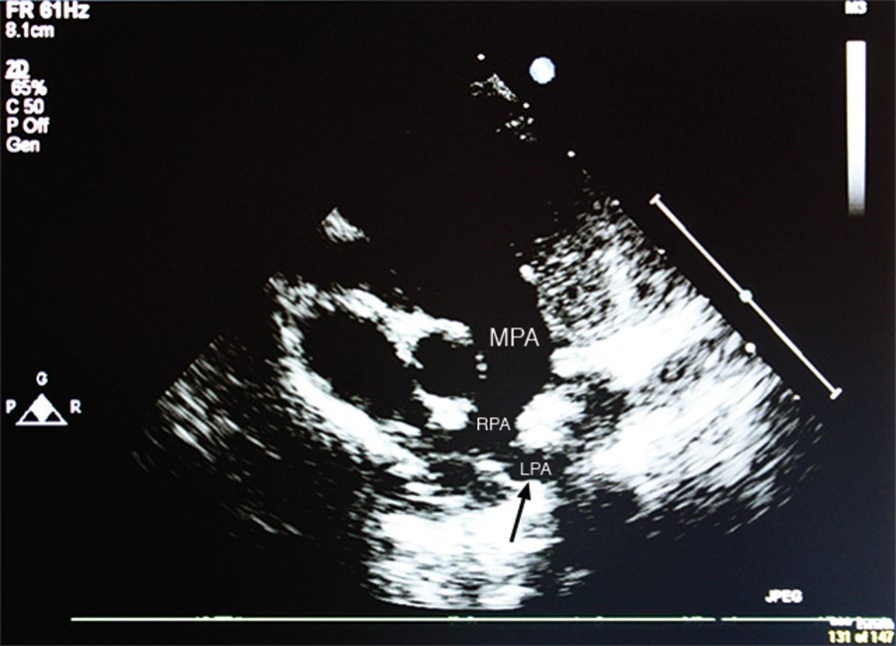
Transthoracic echocardiogram showing main pulmonary artery (*MPA*) giving rise to right pulmonary artery (*RPA*) with arrow depicting left pulmonary artery (*LPA*) arising from its distal segment
